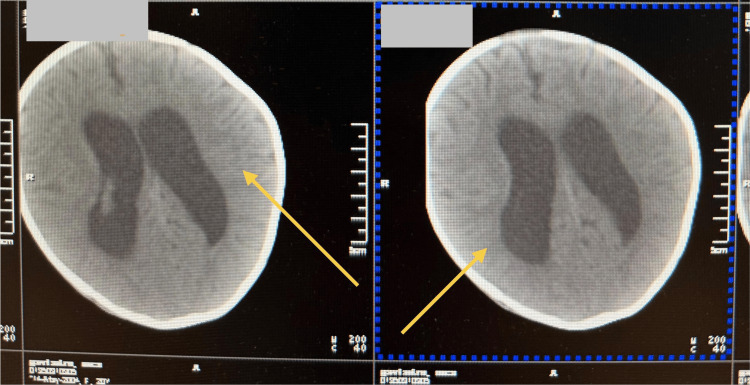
CT of the head showing ventriculomegaly CT: computed tomography
